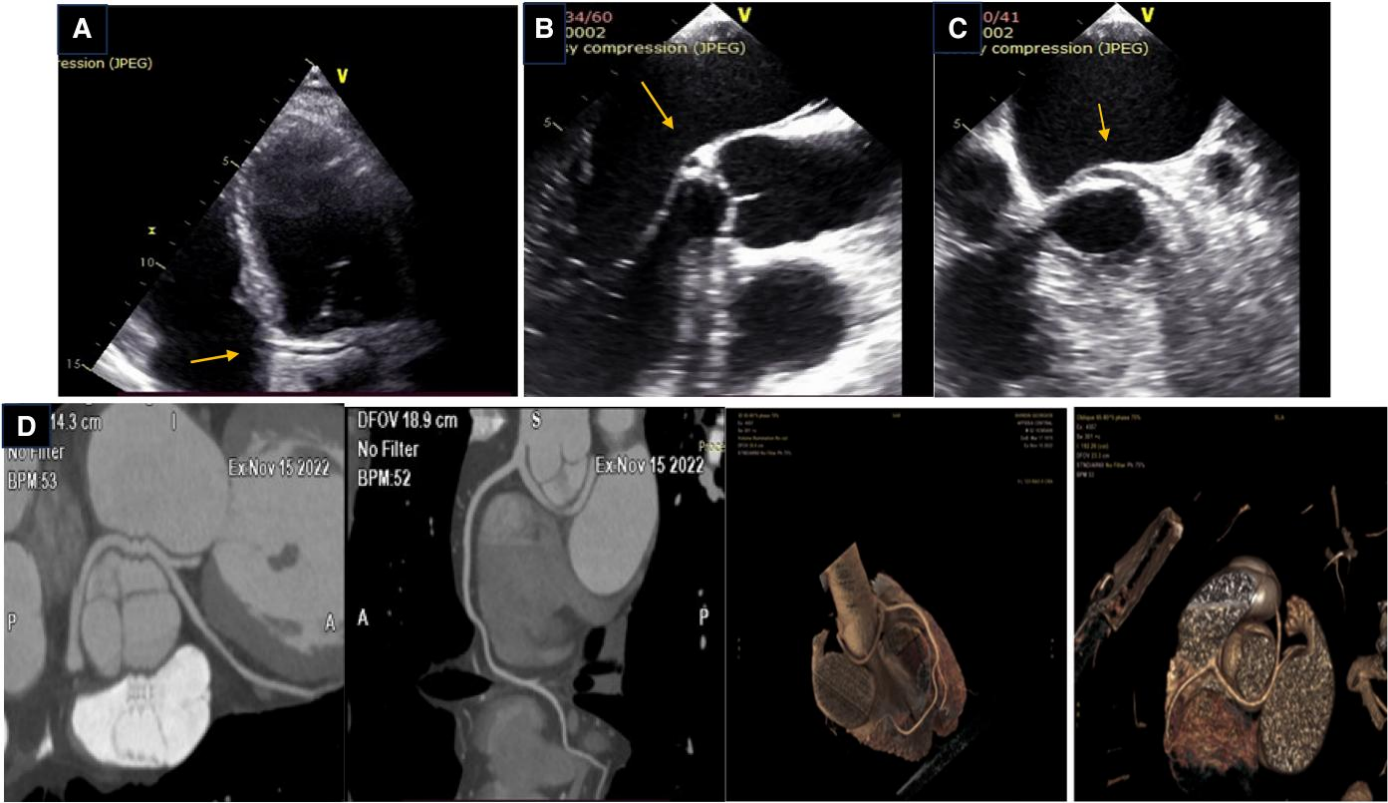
(*A*) Transthoracic echocardiography. Apical 5-chamber view. ‘RAC’ sign—a linear structure with vascular characteristics crossing transversely to the aorta (arrow). (*B*) Transoesophageal echocardiography. Mid-esophageal view at 120°: a circular structure below the non-coronary cusp, corresponding to the ‘bleb’ sign. (*C*) Transoesophageal echocardiography. Mid-esophageal view at 50°; the aorta is shown in short axis. A vascular structure with a retroaortic course is observed (arrow). (*D*) Coronary Computed Tomography Angiography. 2D slices and 3D reconstruction of the retroaortic course of the left main (LM) and the left coronary artery originating from the proximal right coronary artery.
